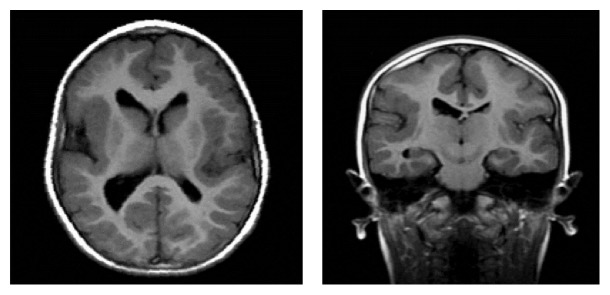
MRI scans. Cortical dysplasia.
